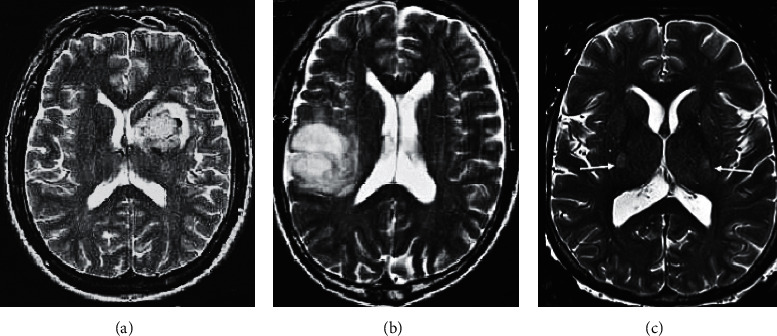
MRI images of some subjects. (a) MRI image of a female patient. (b) MRI image of a male patient. (c) MRI image of a patient in CG.
